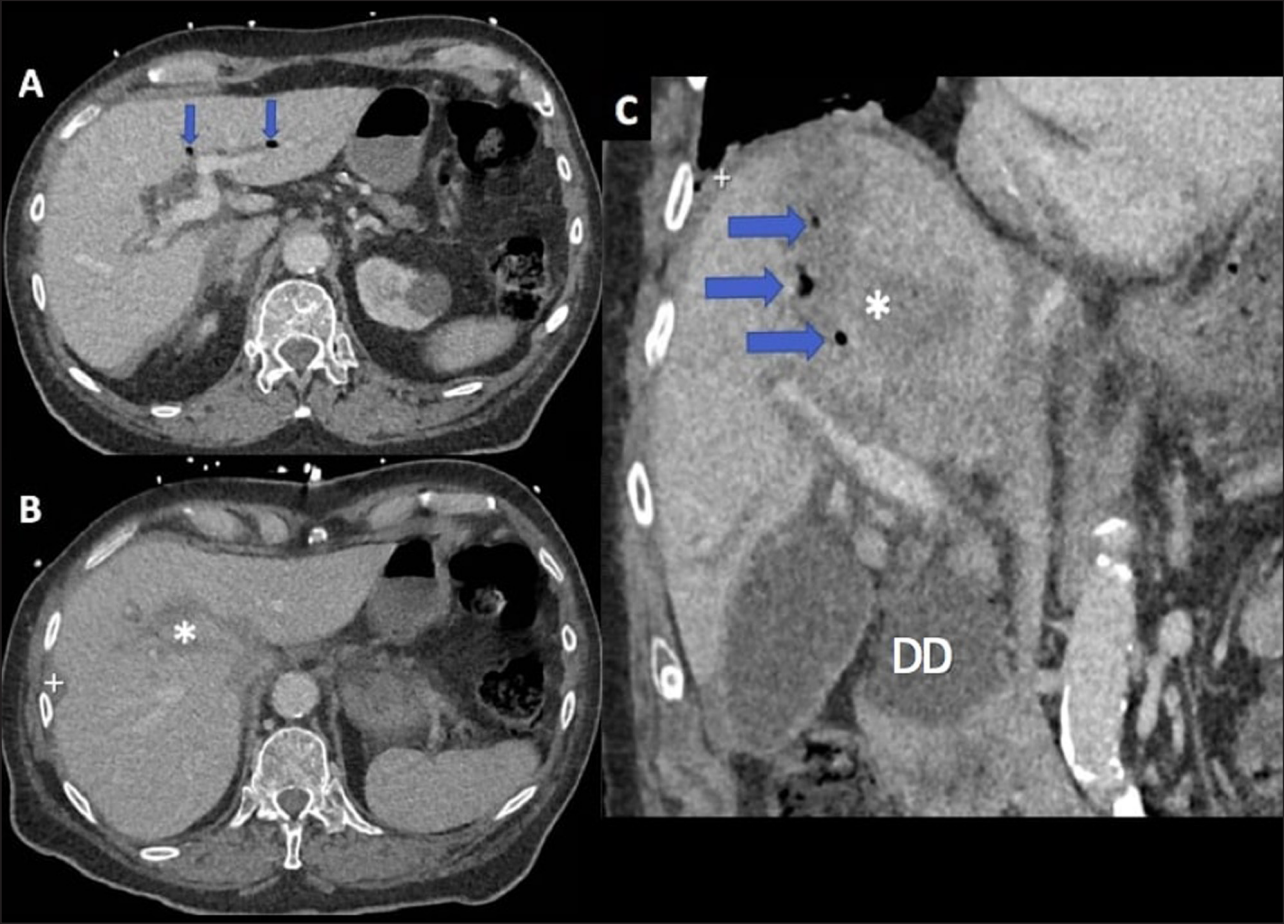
The patient was conservatively managed in intensive care unit. The clinical and radiological outcome was good, with progressive regression of hepatic traumatic lesions and pneumobilia until the last follow-up CT at day 75 (Figure 2).
